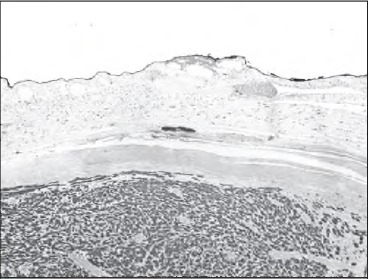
Computed tomography showing a 5cm submucosal homogenous lesion arising from the fundus of the stomach
